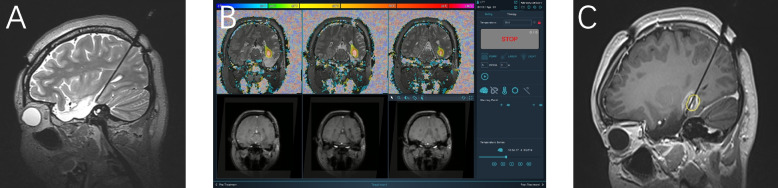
Patient 7: A 24-year-old female still has epileptic seizures after left temporal lobectomy, with approximately 15 seizures per month. It is considered that there is a residual epileptogenic lesion in the medial posterior temporal lobe. A Before ablation, T2-weighted interoperative MRI showed a trajectory implanted in the medial posterior aspect of the left temporal lobe. B Monitoring planes (coronal position) are determined for the gradient echo scan (GRE) needed for thermal imaging. The high-quality T1-weighted image is overlaid onto the near-real-time thermal and tissue damage calculations. The colored areas in the image represent different temperatures and can be used for real-time temperature measurement. C After ablation, T1-enhanced-weighted MRI showed circular enhancement in the posterior temporal lobe, indicating the ablation range
